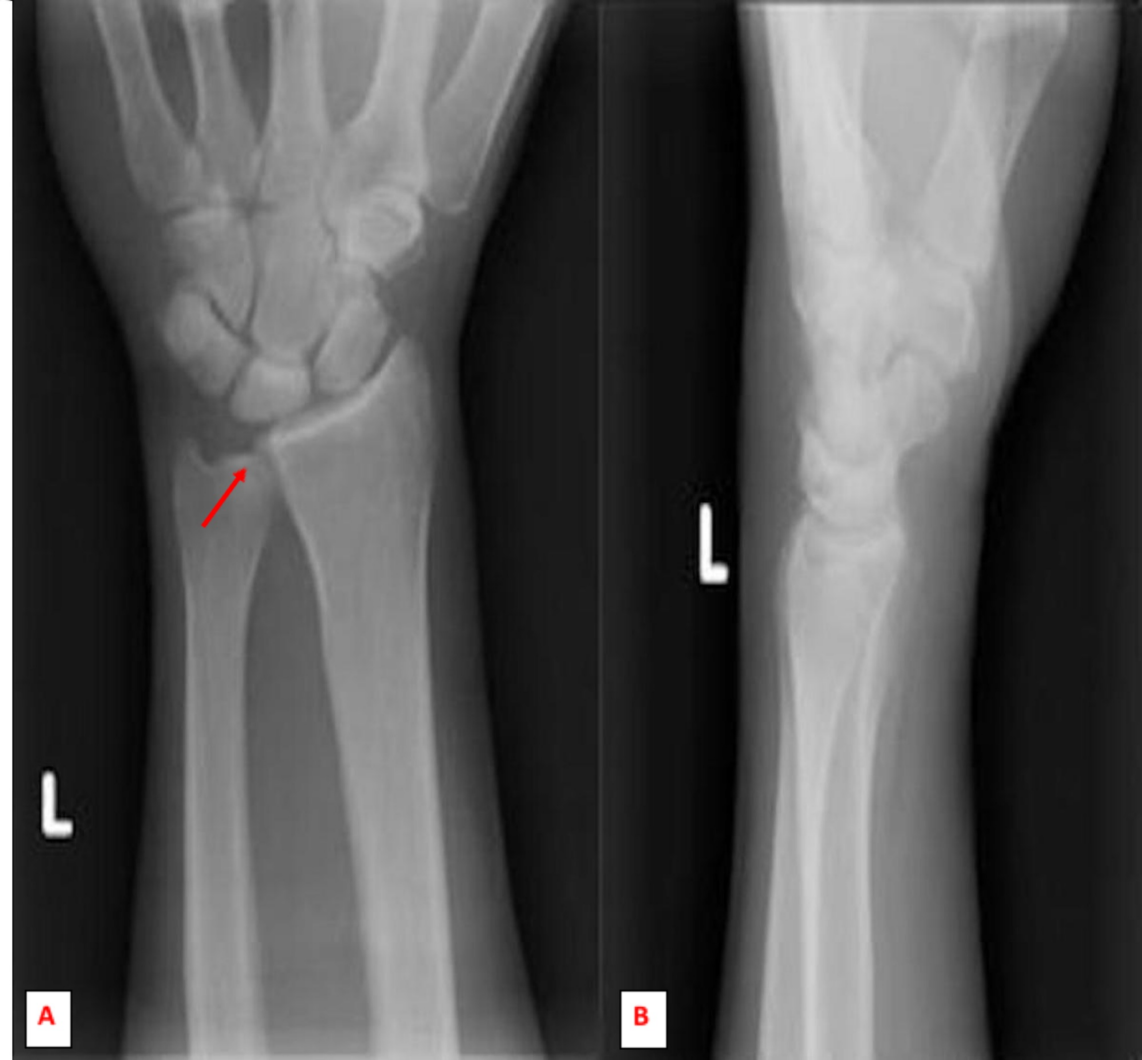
Initial anterior-posterior (A) and lateral (B) radiographic imaging of the left wrist showed normal alignment, articular surfaces and preserved spaces. No obvious lytic or blastic lesions. A negative ulnar variance noted.
